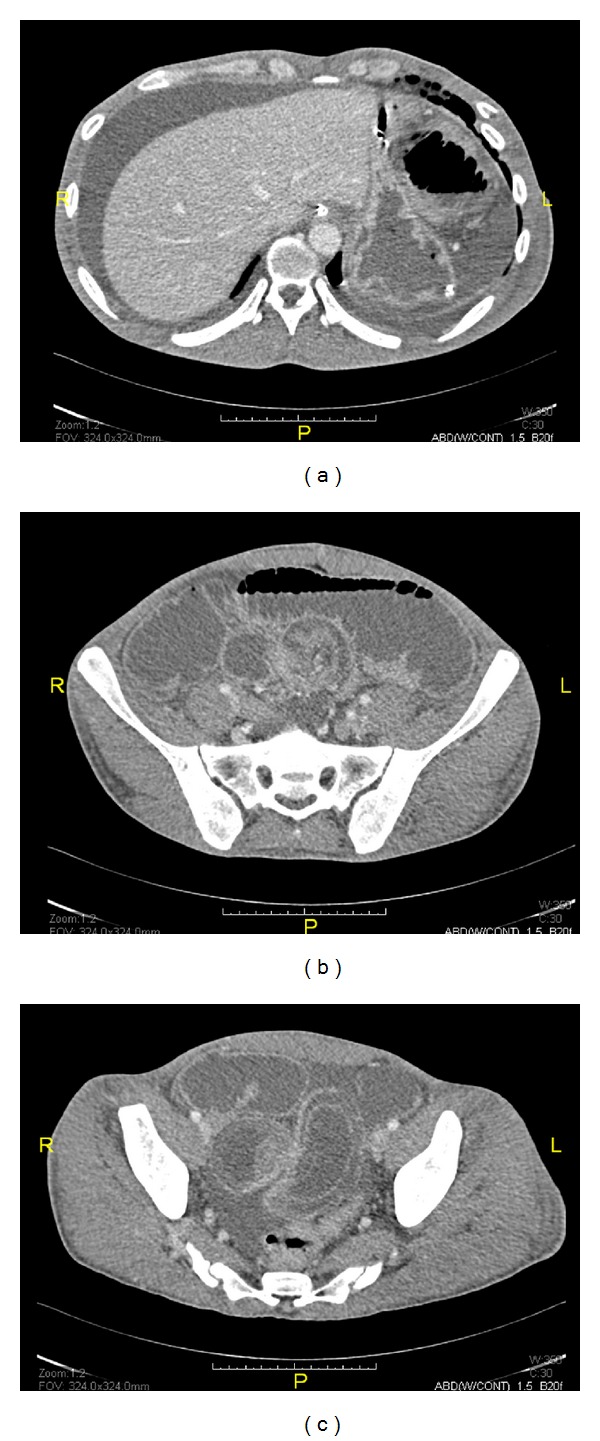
Abdominal CT scanning on day 9 of admission (day 5 after symptoms) showing peritoneal effusion, severely dilated small bowel, and suspected intussusception of the distal Ilium.
